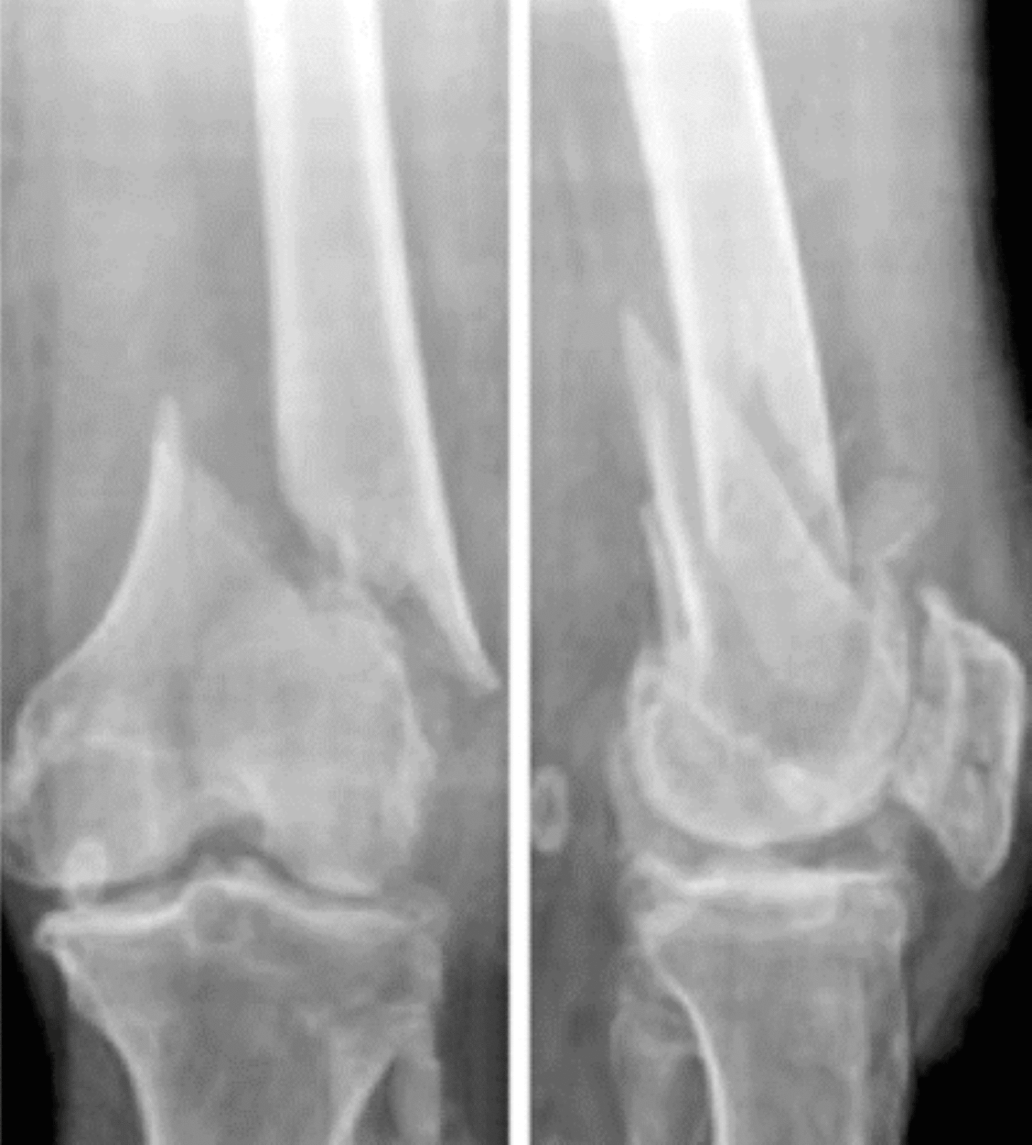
Case 2 preoperative X-rays: anteroposterior and lateral views
